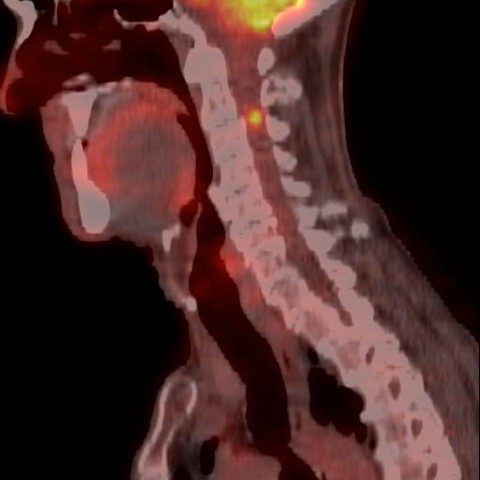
Sagittal fused PET-CT scan of his cervical spine showing a small focus of intense tracer uptake in the spinal canal at C2-C3 level in his neck.
